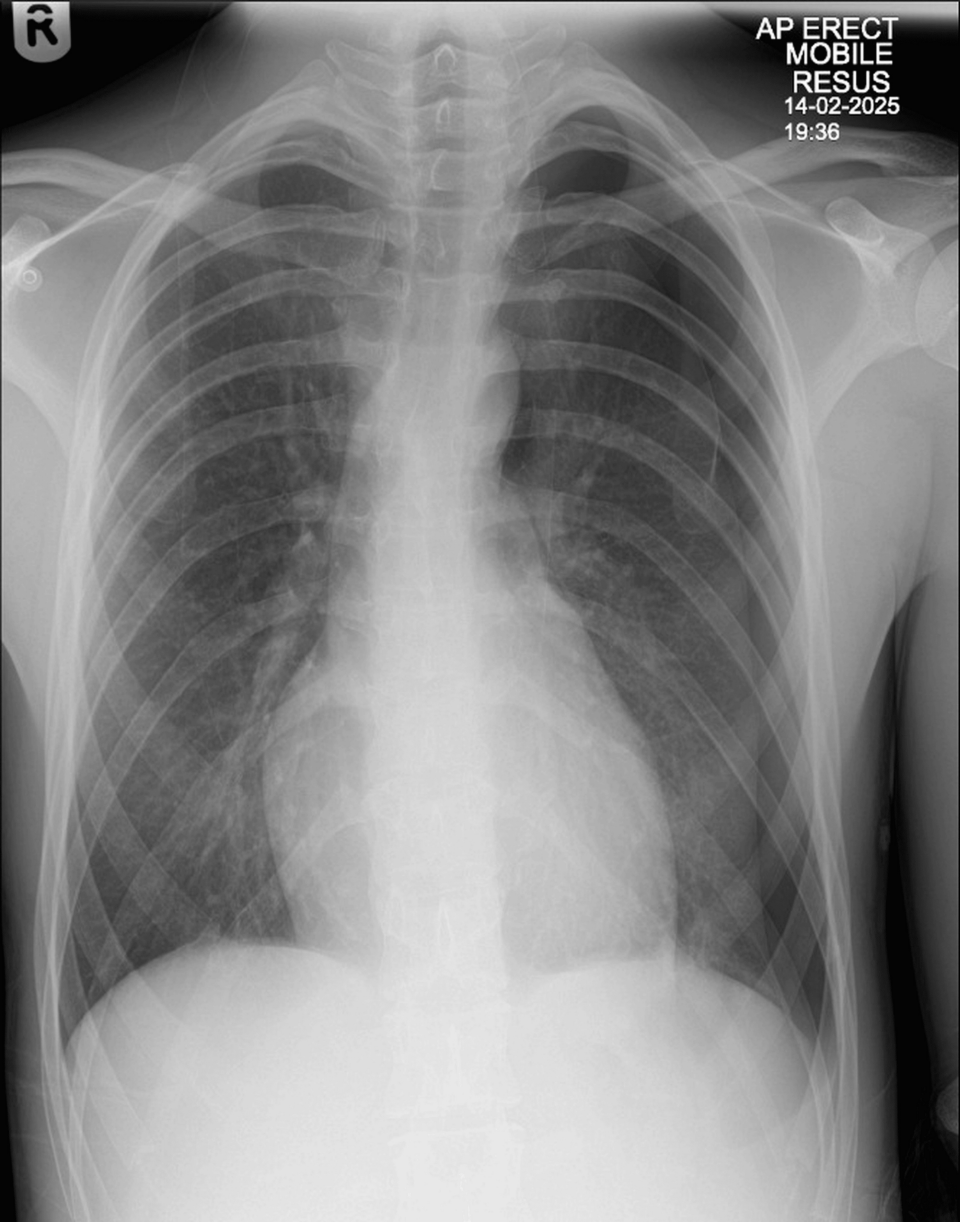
CXR following needle aspiration demonstrating enlargement of pneumothorax and partial lung collapse. CXR, chest X-ray
